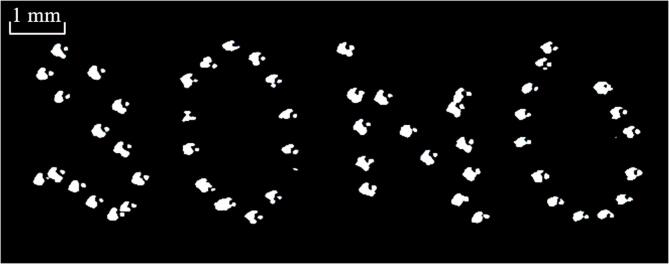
Bubble trajectory (‘SONO’, the acronym for *Ultrasonics Sonochemistry*) extracted from the experimental results.
